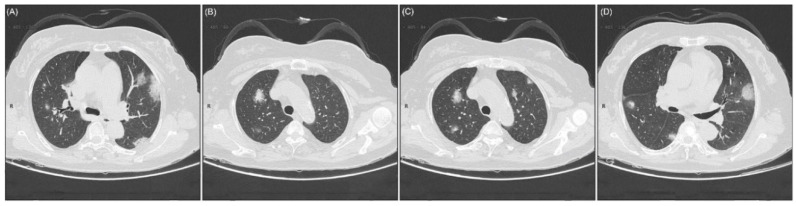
Atypical CT imaging of a 56-year-old female presented with a history of 3 days of persistent fever. Laboratory findings: reduced total protein level (54.0 g/L), reduced albumin value (35.5 g/L), low value of globulin (18.5 g/L), white blood cells (WBCs) (4.87 × 10^9^/L), lymphocytes (0.49 × 10^9^/ L), and decreased level of eosinophil (0 × 10^9^/L). Imaging inspection: (A) A lateral section of the center of right lung lobe covered GGO. (B) Patchy GGO in the upper part of the right lung with sporadic consolidated lesions. (C) GGO in both lungs with sporadic consolidation lesions. (D) Uneven GGO in the middle and dorsal section of the right lung. Adopted from Kui et al. through open-access license [69].
